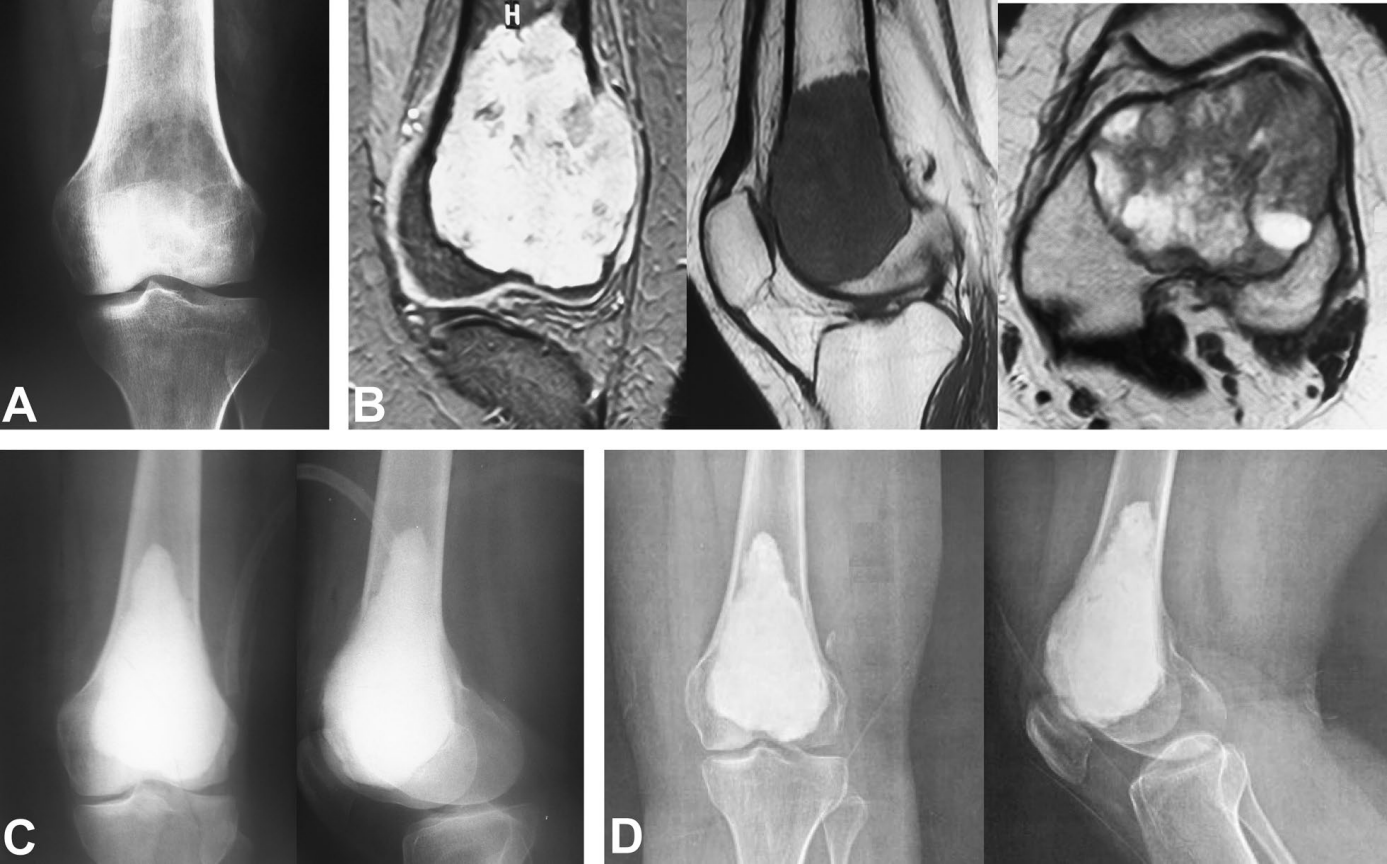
A 25-year-old female with giant cell tumor of the distal femur treated with curettage and cementation. A Preoperative AP X-ray. B Preoperative coronal, sagittal, and axial MRI cuts. C Immediate postoperative AP and lateral X-rays. D Ten-year follow-up AP and lateral X-rays with KL grade 1
